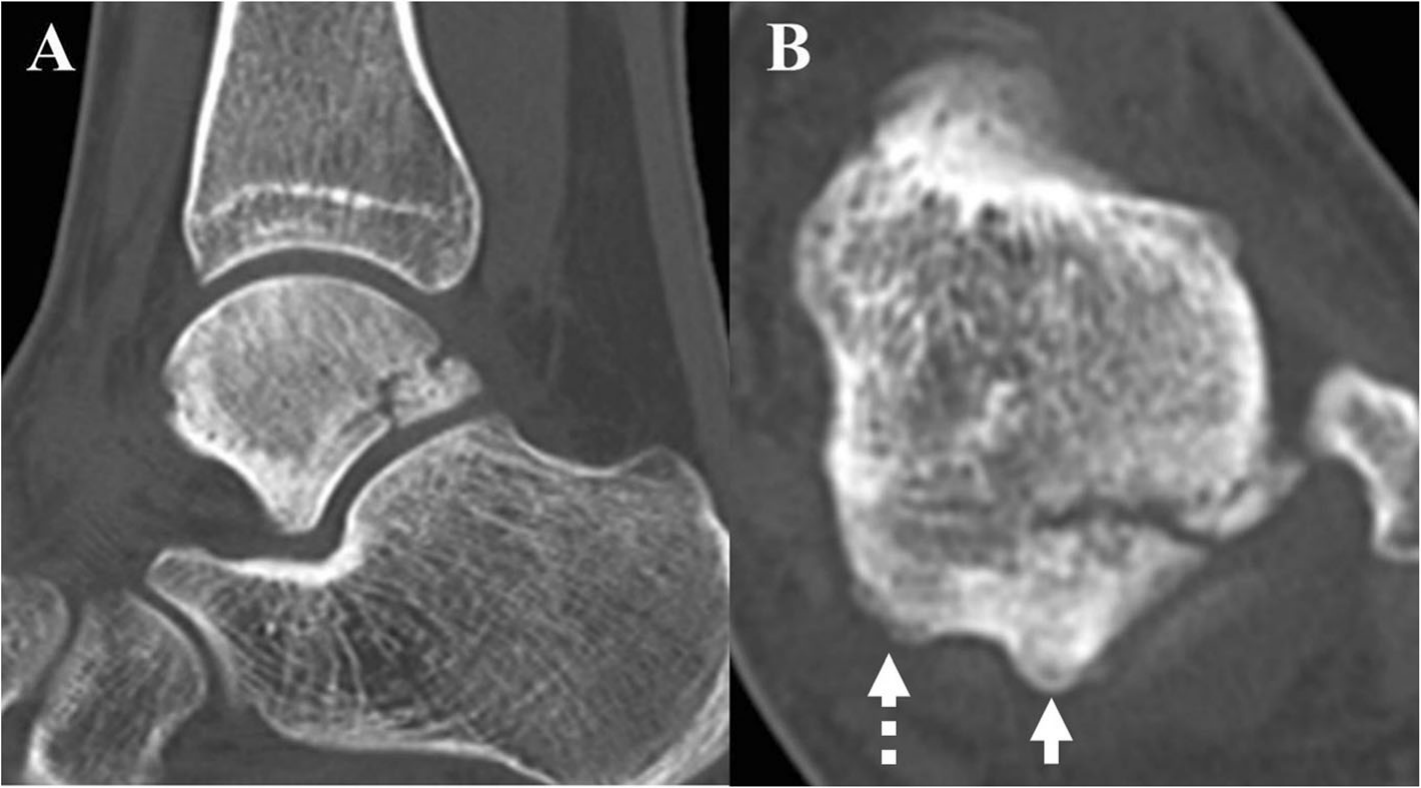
Preoperative non-contrast CT scan. a Sagittal and (b) axial views showing the fracture line located just lateral to the groove for flexor hallucis longus (FHL) tendon at a level just proximal to the subtalar joint. Posterior process of the talus comprising the medial (dotted arrow) and lateral (arrow) tubercles, separated by the groove for the FHL tendon. CT, computed tomography
